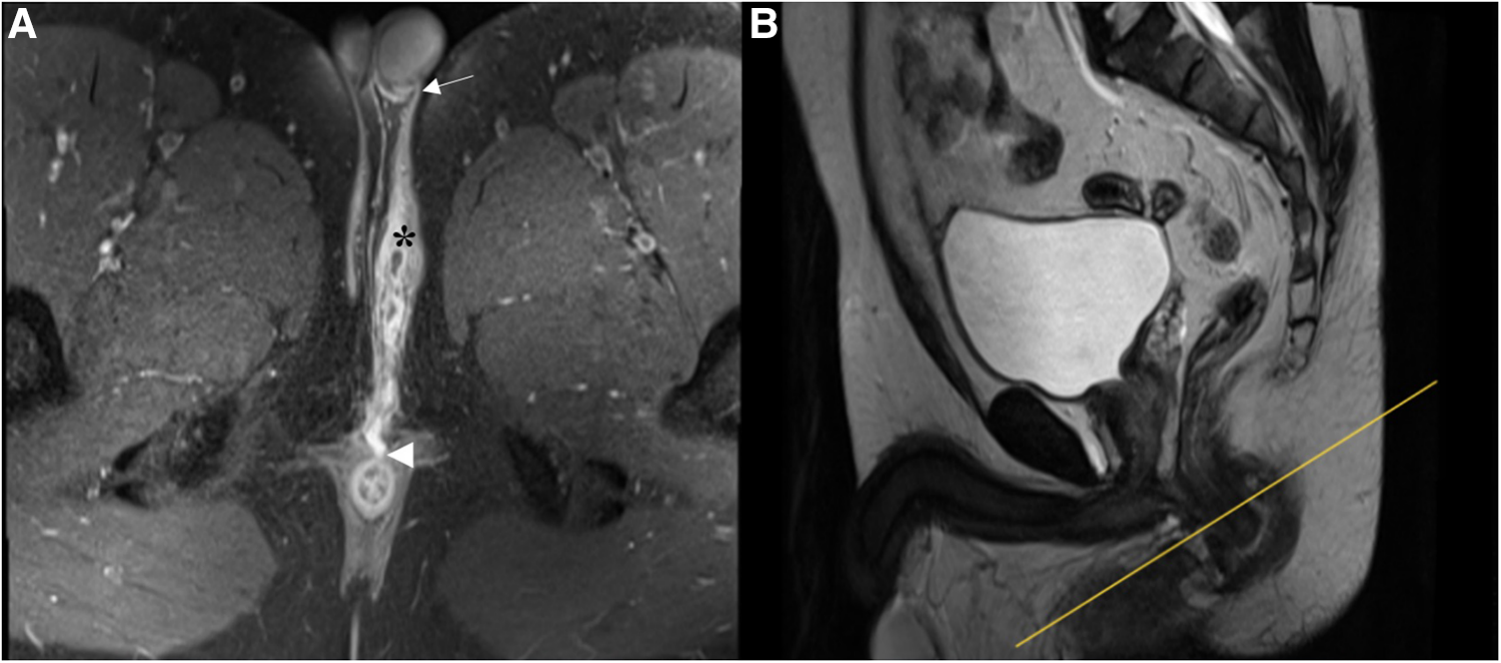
A low transsphincteric fistula extending to the left scrotum. (A) Post-contrast FS axial T1-weighted image shows a fistula (asterisk) extending into the left scrotum (arrow) and opening radially into the anterior wall of the anal canal at 12 o'clock position (arrowhead). (B) Sagittal T2-weighted image shows the corresponding location (yellow line) where the fistula penetrating the external sphincter. The fistula traversed the lower half of the anal canal and was classified as low transsphincteric.
